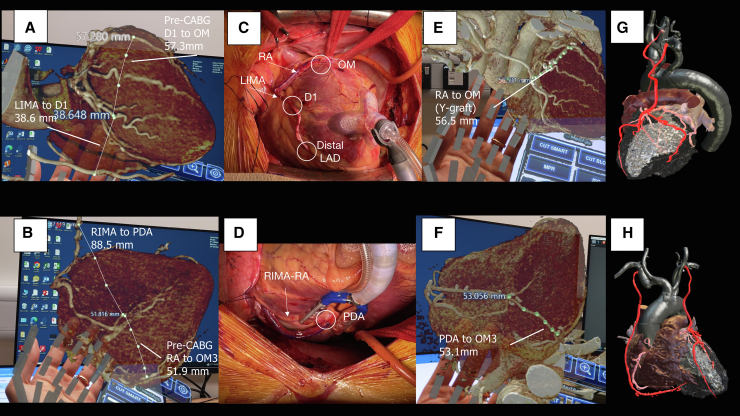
A and B, Show the 3D hologram derived from the pre-CABG CT scan with the measurements of the distance between the LIMA to D1, D1 to OM1, and RIMA to PDA to OM3 displayed. The distance between D1 to OM1 and PDA to OM3 corresponds to the length of RA grafts needed to be harvested. C and D, Show the intraoperative photographs of the LIMA, RIMA, and the 2 RA grafts. E and F, Show the lengths of the implanted RA grafts measured on the post-CABG CT hologram, which is quite similar to the pre-CABG planned lengths. G and H, Show the postprocedure 3D-reconstructed images for 3D printing preparation. *LIMA*, Left internal mammary artery; *CABG*, coronary artery bypass graft surgery; *OM1*, first obtuse marginal branch of the left circumference artery; *RIMA*, right internal mammary artery; *PDA*, posterior descending artery; *RA*, radial artery; *OM3*, third obtuse marginal branch of the left circumference artery; *LAD*, left anterior descending artery; *3D*, three-dimensional; *CT*, computed tomography; *D1*, first diagonal branch of the left anterior descending artery.
